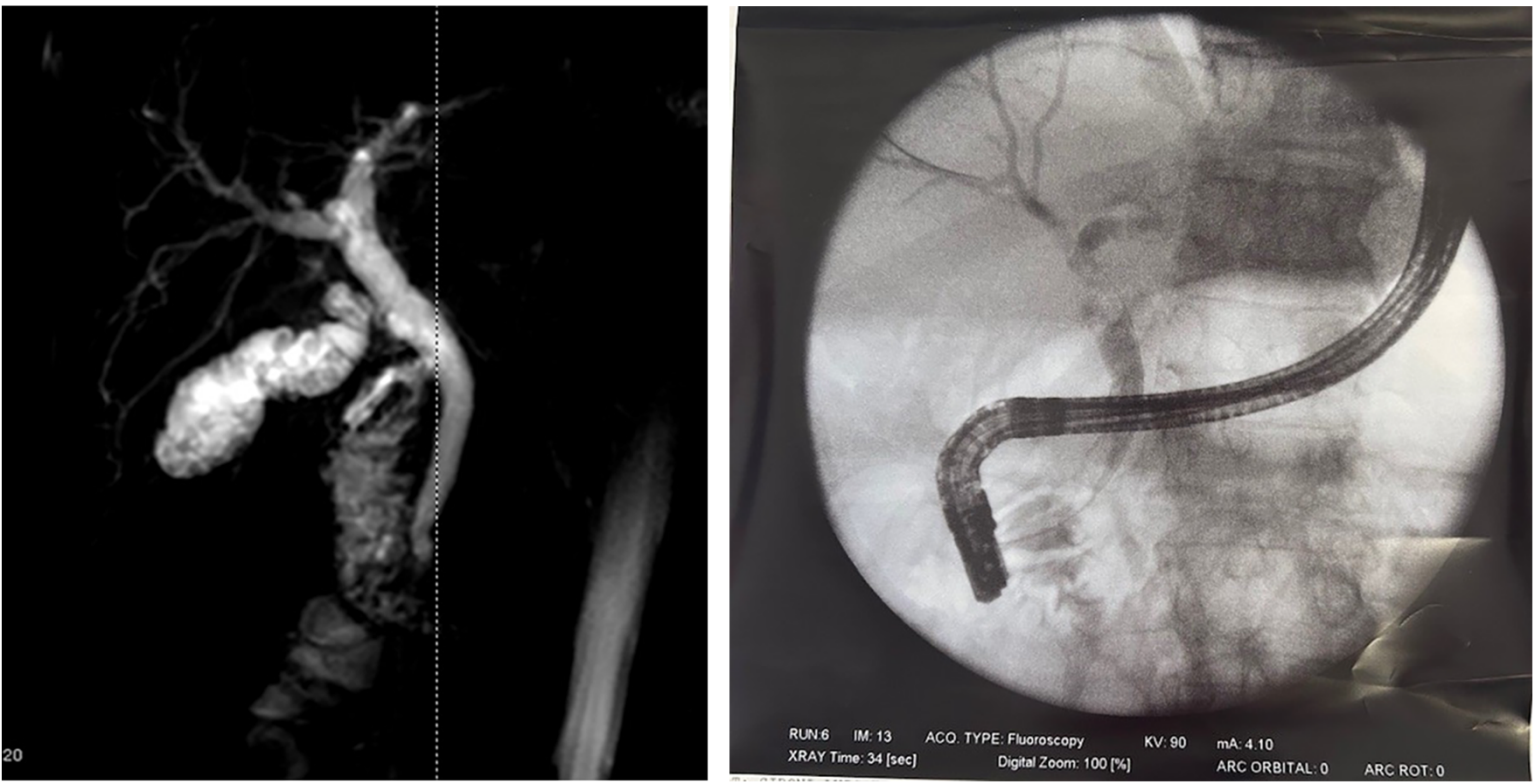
Cholecysto-choledocholithiasis diagnosed at the MRI and Endoscopic retrograde cholangiopancreatography image.
